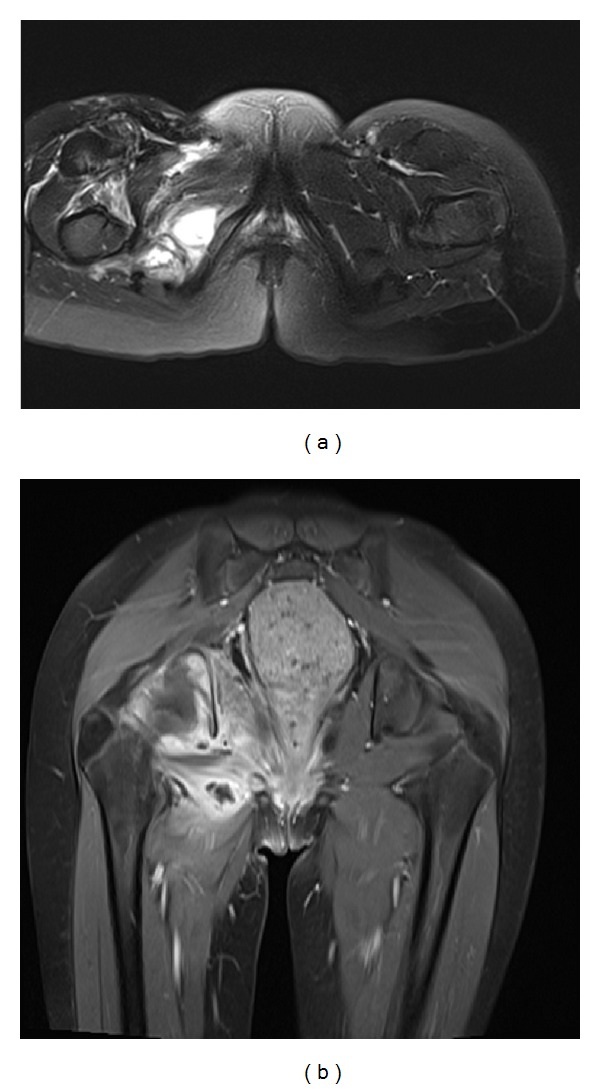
Axial T2-weighted (a) image, with fat saturation, showing right hip effusion and extensive myositis. A four weeks later (b) coronal T1 image with fat saturation, after gadolinium, showing increased signal intensity in the right femoral head, with erosions. There was associated edema of the surrounding tissues, hip effusion and myositis with abscess.
